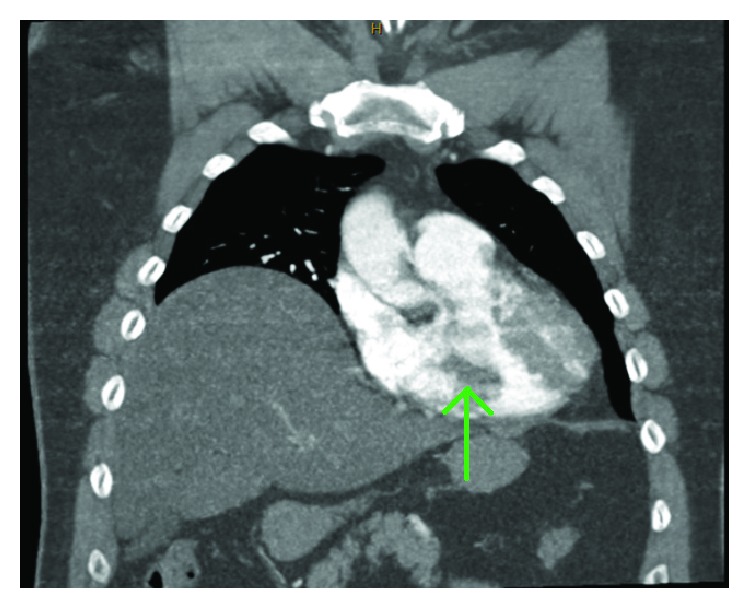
Computed tomography pulmonary angiogram revealed a broad-based filling defect in the right ventricle (green arrow), with incidental finding of preoperative raised right hemidiaphragm.
